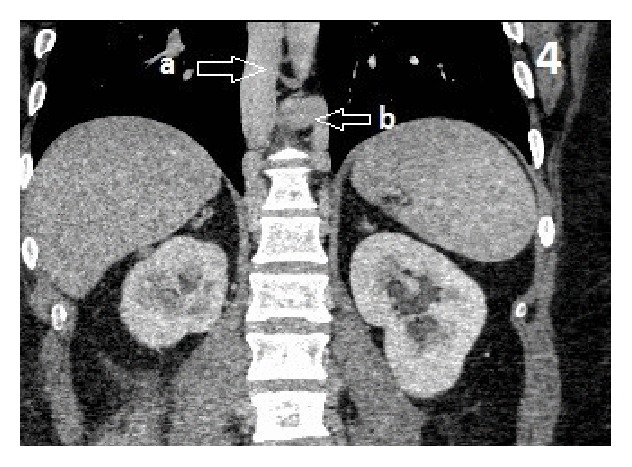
Abdomen CT coronal view: (a) enlarged azygous vein, (b) enlarged hemizygous vein.
